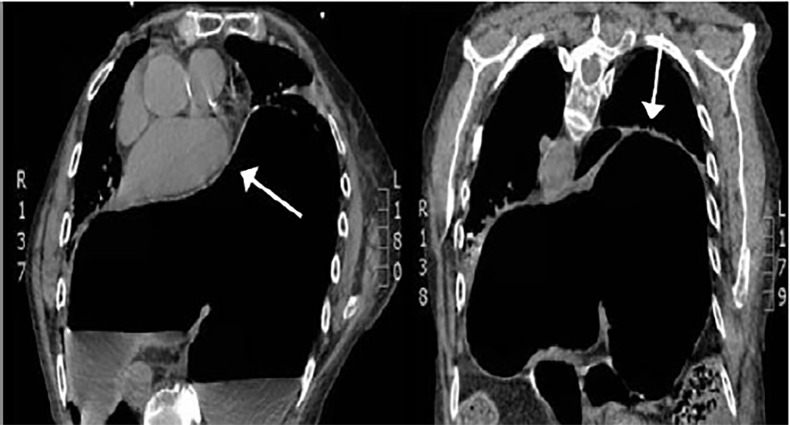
Computed tomography (CT) of the chest. Left: Axial view of a CT chest demonstrating gastrointestinal (GI) contents in the thoracic cavity with cardiovascular compression (white arrow). Right: Coronal view of a CT chest demonstrating GI contents in the thoracic cavity (white arrow).
